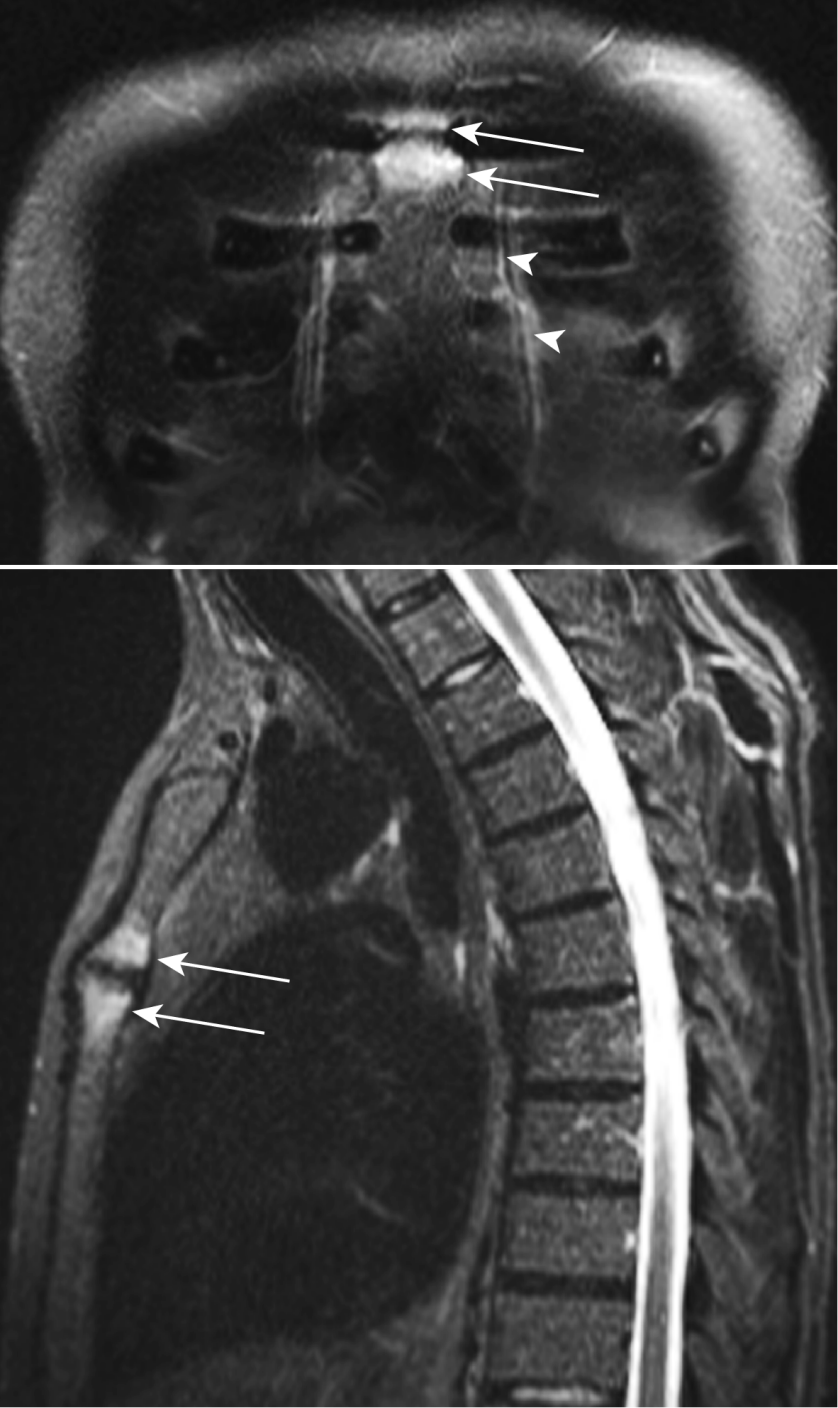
Inflammatory lesions of the anterior chest wall displayed by whole-body MRI in an AS patient complaining about chest wall pain. Bone marrow edema (arrows) in both parts of the manubriosternal joint on coronal and sagittal short τ inversion recovery (STIR) sequences in a 46-year-old human leukocyte antigen B27 (HLA-B27)-positive male patient with ankylosing spondylitis and anterior chest wall pain. He had a 6-year history of inflammatory back pain. The arrowheads point to the mammary veins.
